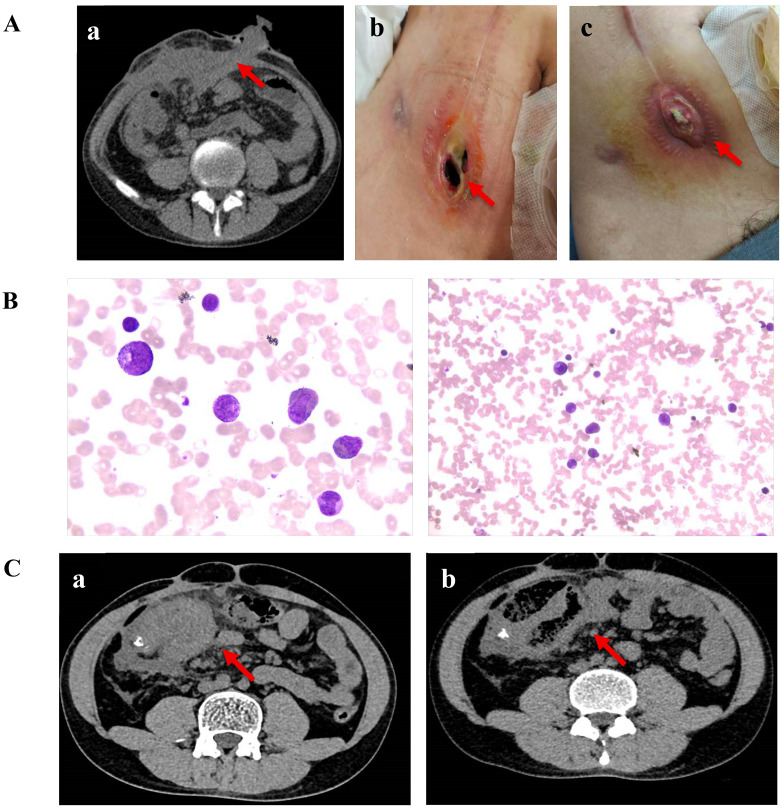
(A) (a) Postoperative CT imaging, (b) Postoperative wound appearance, with the arrow indicating an associated sinus tract in the right lower quadrant, (c) Wound appearance after treatment, showing substantial wound healing. (B) Bone marrow examination demonstrates granulocytic maturation arrest, characterized by an increased proportion of myeloblasts and promyelocytes and a marked reduction in mature granulocytes. (C) The two images show a comparative CT examination: (a) CT scan obtained before treatment with efbemalenograstim alfa, showing significant intestinal wall edema, (b) CT scan after 2 months of using efbemalenograstim alfa, showing marked resolution of the edema.
